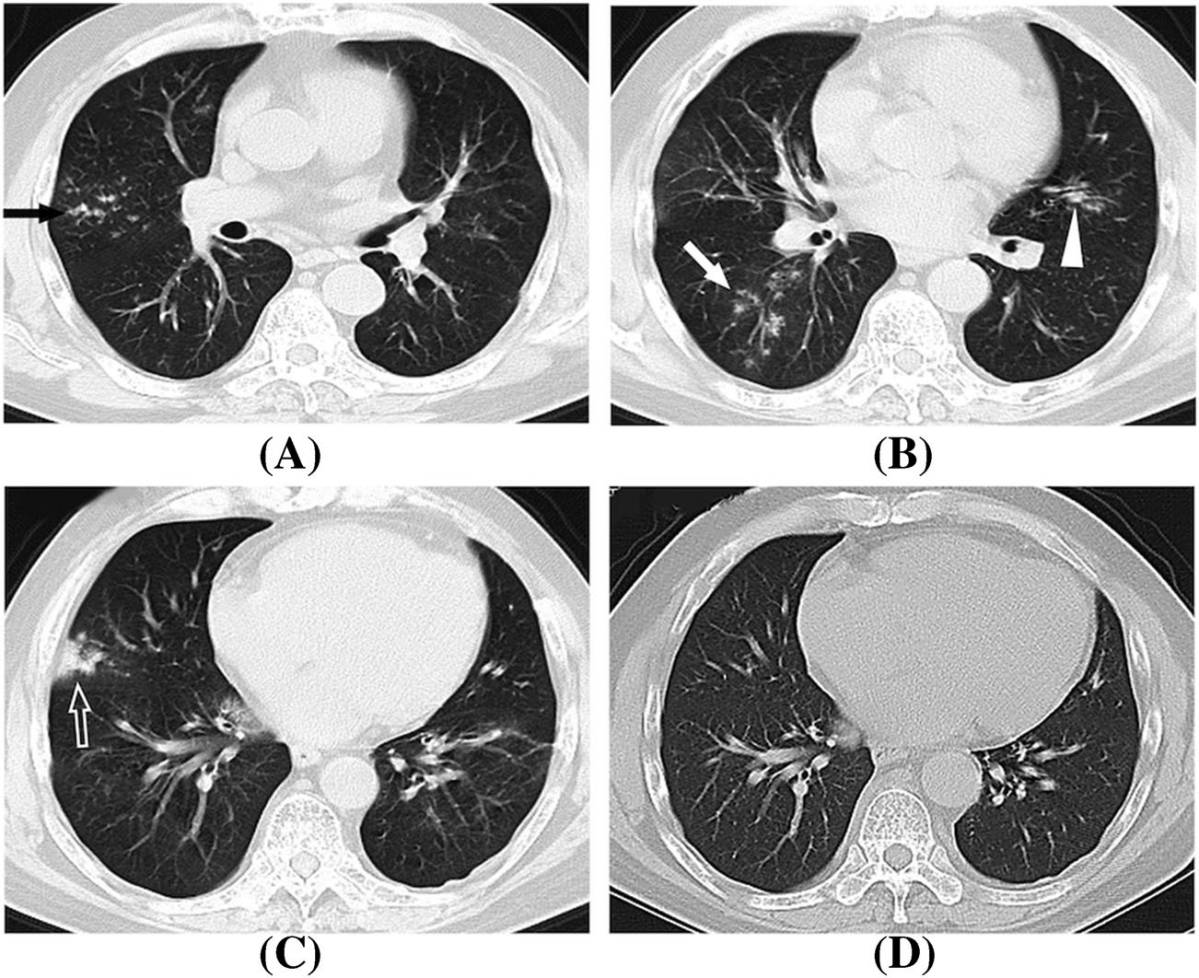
A 52‐year‐old man with severe H1N1 pneumonia, presented with fever and cough. (A–C) Axial CT showed tree in bud nodules (black arrow), centrilobular nodules (white arrow), bronchial wall thickening (white triangle), and focal consolidation (white hollow arrow) with peripheral and central distribution. (D) The lesions were completely absorbed after treatment for 8 days.
